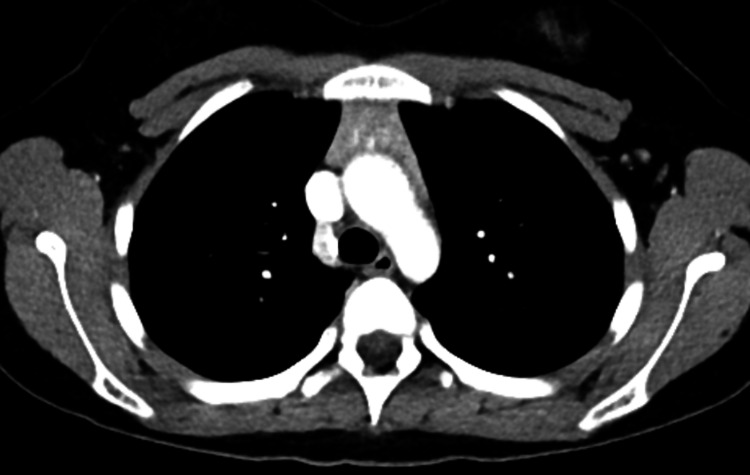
Axial CT chest with IV contrast
